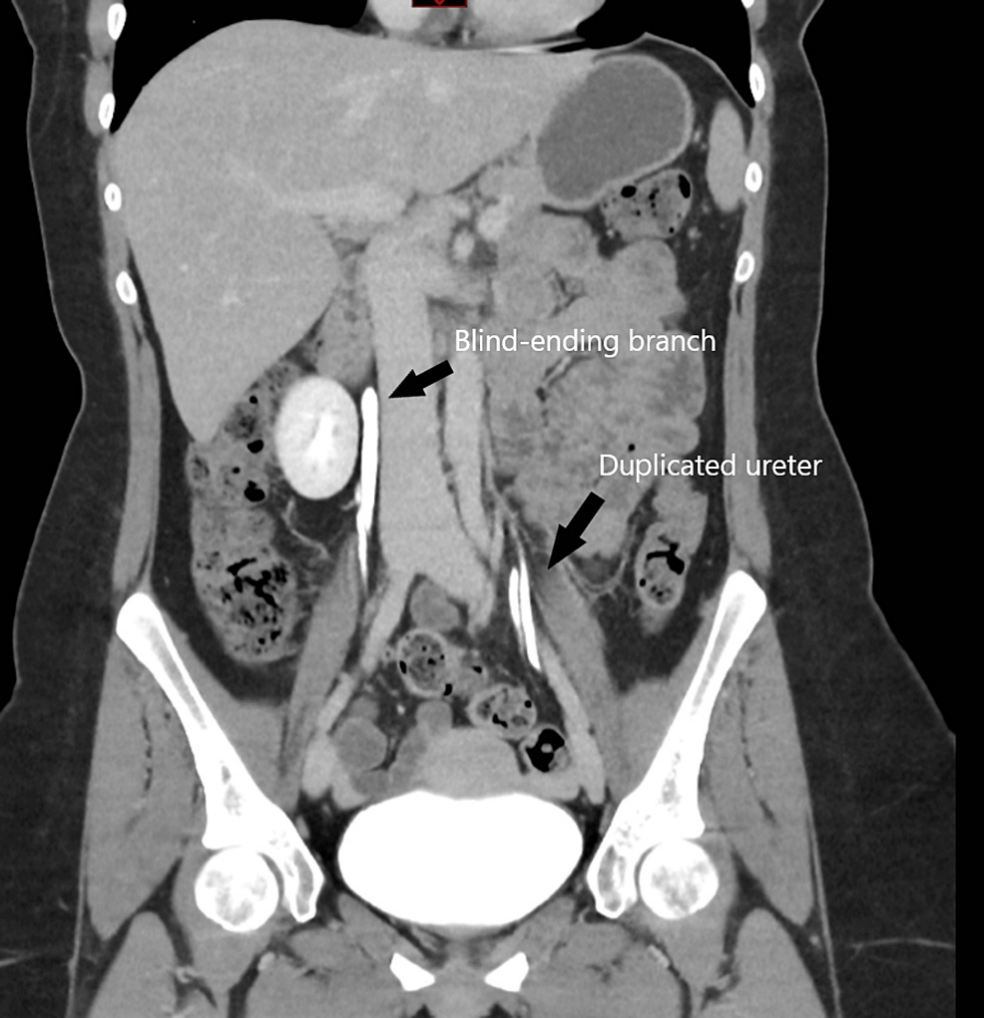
Computed tomography (CT) scan of the urinary tract with contrast showing the blind-ending branch of the bifid ureter on the right side and a duplicated ureter on the left side (sagittal plane).
